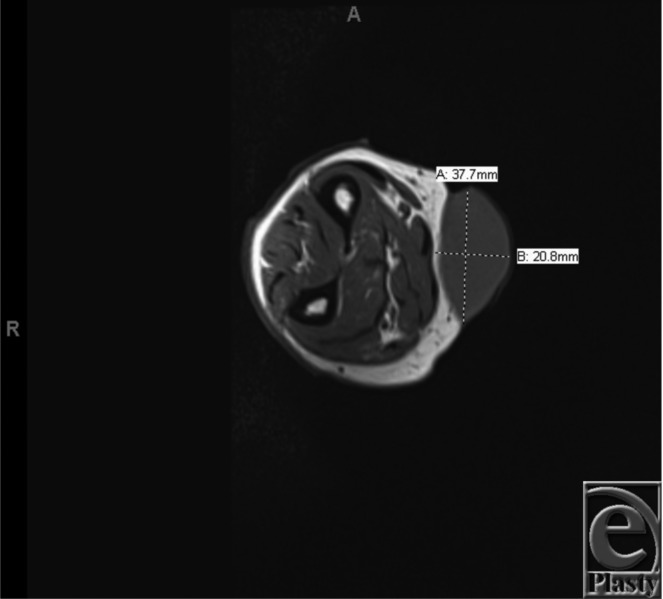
Magnetic resonance image of the right forearm showing the extent of the lesion.
